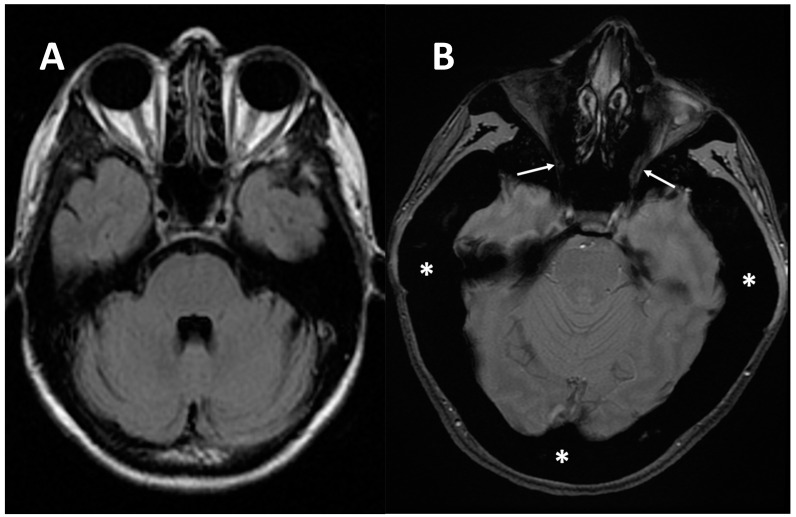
(A) axial MRI of a healthy young female (shown as comparison). (B) axial MRI (T2w*) of a 38-year-old female with a clinical-radiological diagnosis of osteopetrosis (ARO type); narrowing of both optic foramens can be noted (arrows) caused by a diffuse bone thickening. Diffuse bone thickening of cranial bones is associated with a marked ipointense signal intensity of all the skeletal structures related to the diffuse bone sclerosis (asterisks).
